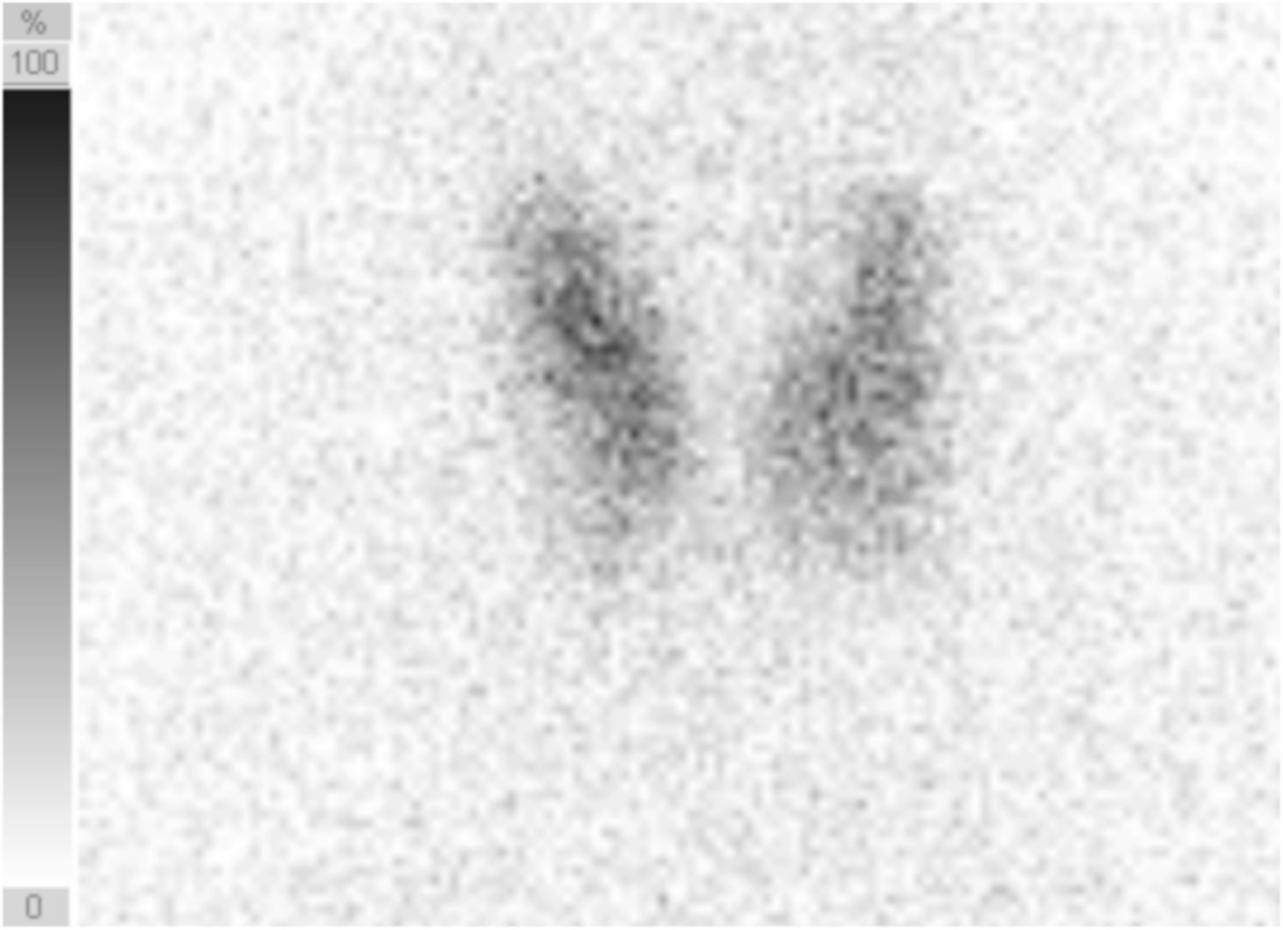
Thyroid gland uptake of ^123^I-scintigraphy. Diffuse uptake of ^123^I by 4.7 % is demonstrated under the thyrotoxicosis condition after 3 h
